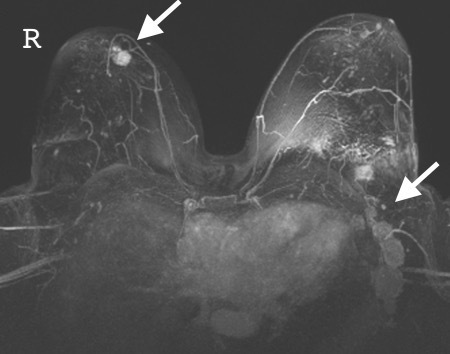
MRI with enhancing primary right breast lesion and axillary lymphadenopathy.
